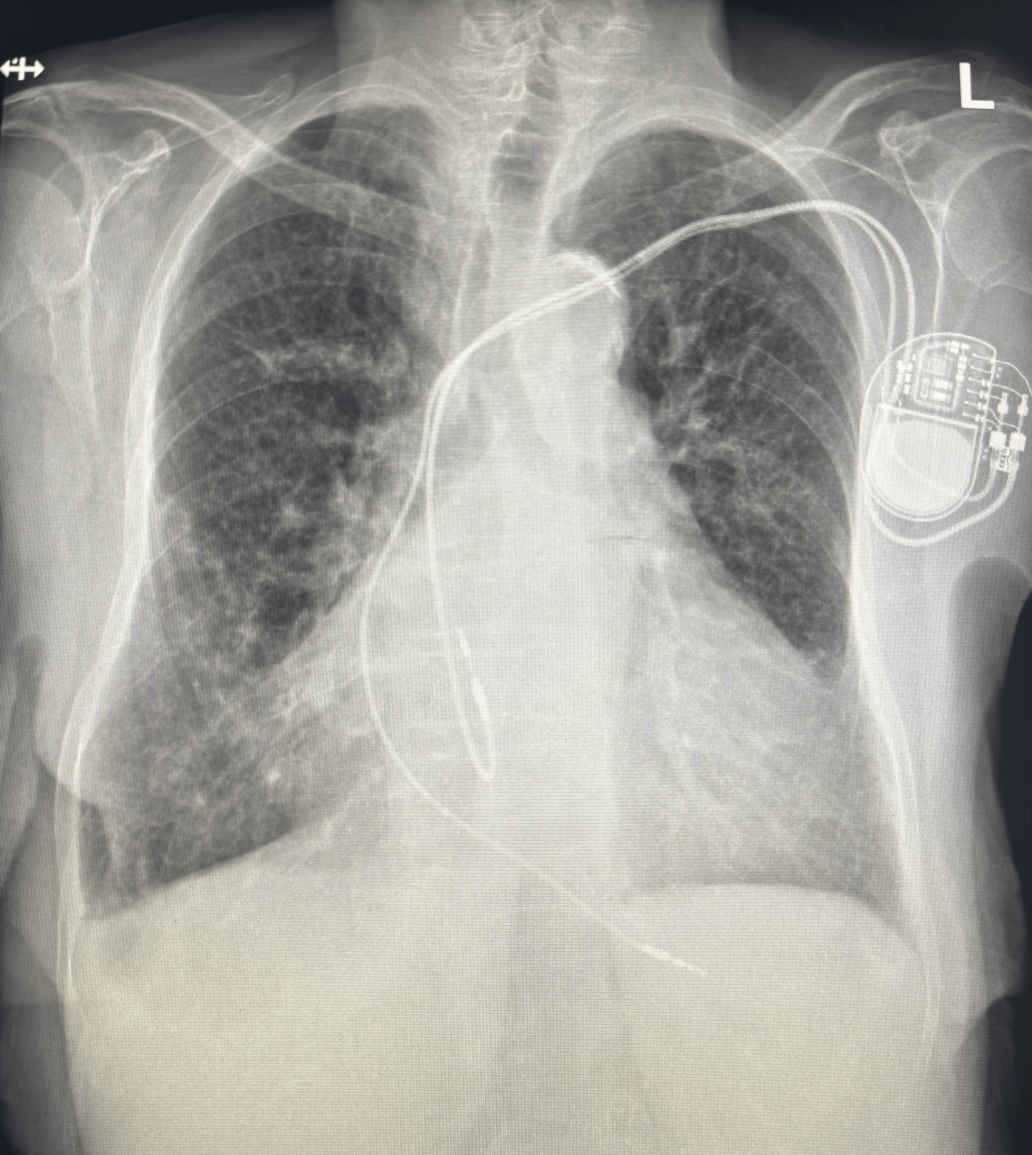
Chest x-ray shows the PC maker in place; otherwise, it is unremarkable for any other pathological findings. PC: pacemaker.
